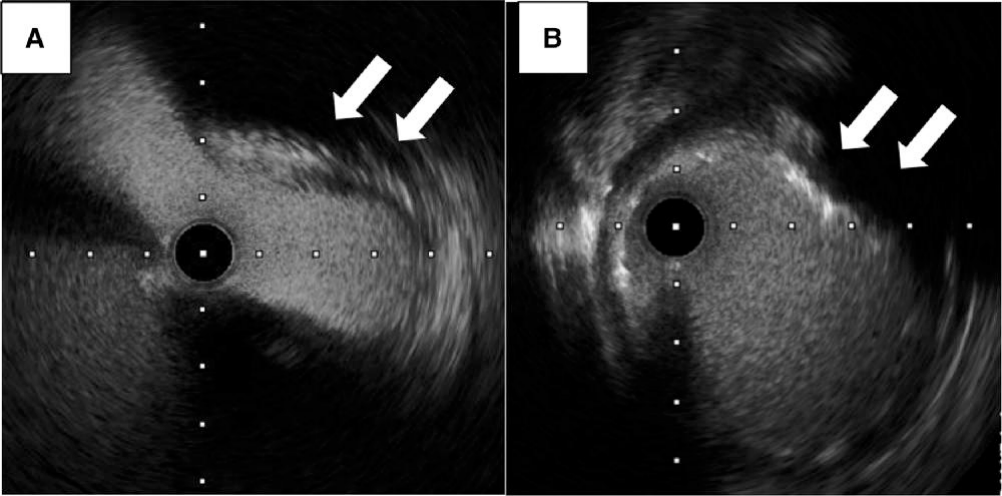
Intravascular ultrasound for examination of the left main trunk compression from outside (white arrows). (*A*) Before percutaneous catheter intervention (PCI) and (*B*) after PCI.
